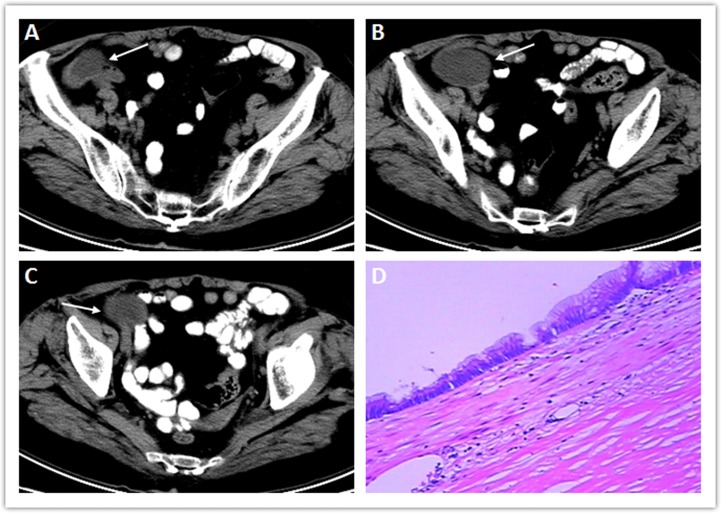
A well-circumscribed tubular cystic mass (arrow) in the right iliac fossa (A–C), displacing the adjacent bowel. The mean CT value of the intratumoral cystic contents was 27 HU. No significant solid component inside the mass was shown on CT. Mucosa reduced to single layer of flat mucinous epithelium with apical mucin droplets and pseudostratified hyperchromatic nuclei (D).
